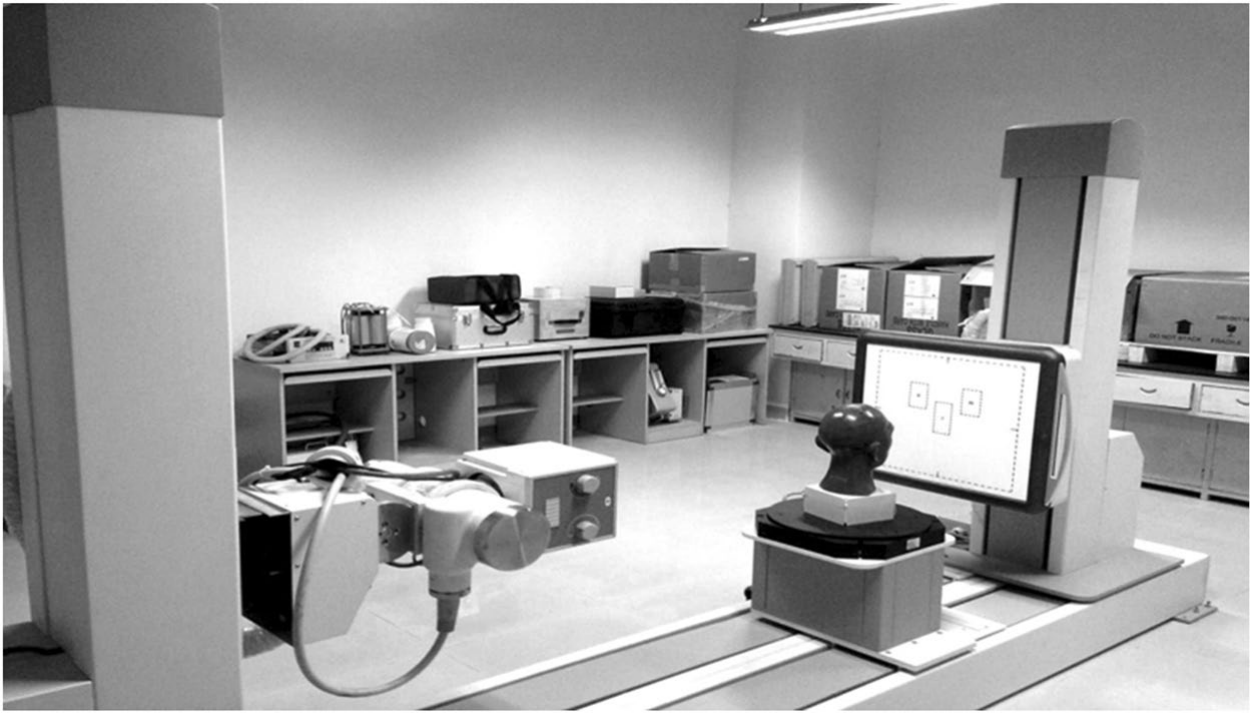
In-house bench-top CBCT system for raw projection acquisition.
